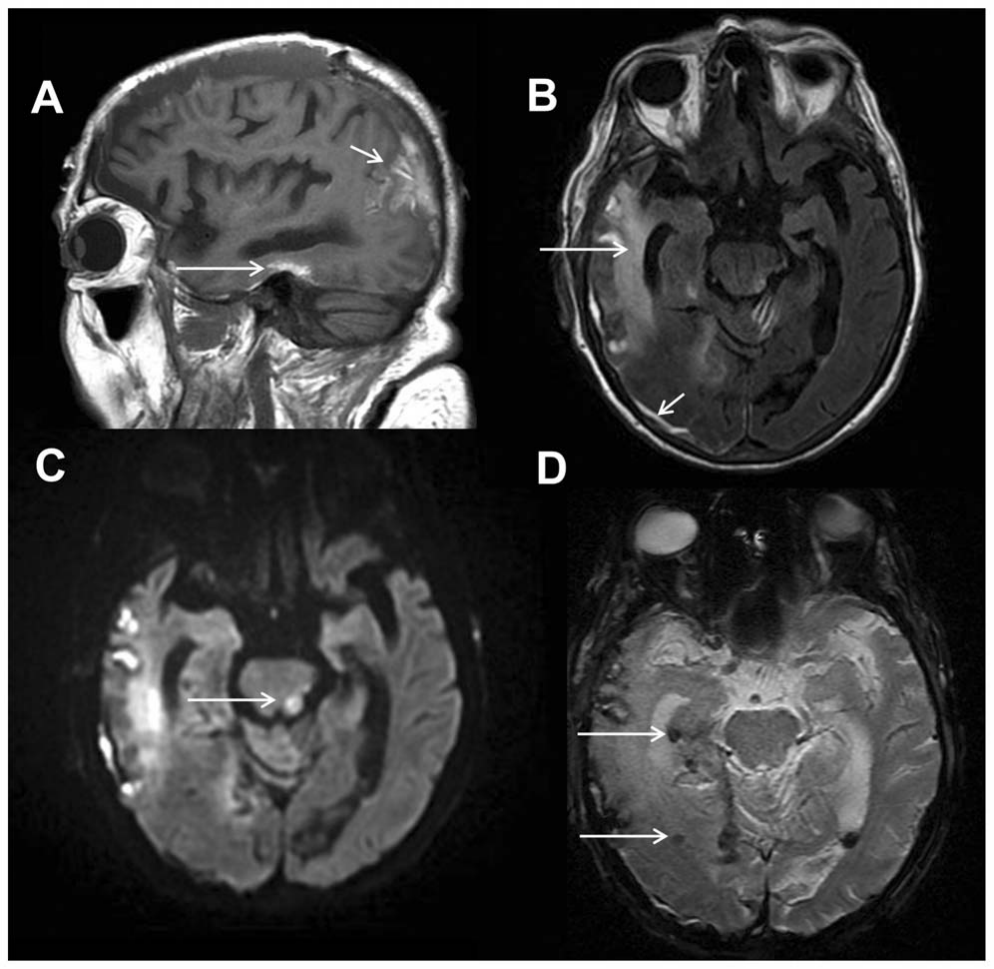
Intracranial hemorrhages and diffuse axonal injury. 83 year old female status post fall. Sagittal T1-weighted image (A) shows hyperintense signal involving the lateral right temporal lobe consistent with cortical contusion and subacute blood products (white arrow). Axial T2-weighted FLAIR image (B) depicts edema in the right temporal lobe from traumatic contusion (long white arrow) and a subdural hemorrhage overlying the lateral right temporal and occipital lobes (short white arrow). Axial diffusion weighted trace image (C) shows a focus of restricted diffusion within the left dorsolateral midbrain compatible with non-hemorrhagic diffuse axonal injury (white arrow). Axial gradient recalled echo image (D) shows a punctate focus of susceptibility effect within the right temporal lobe compatible with hemorrhagic diffuse axonal injury (white arrow).
